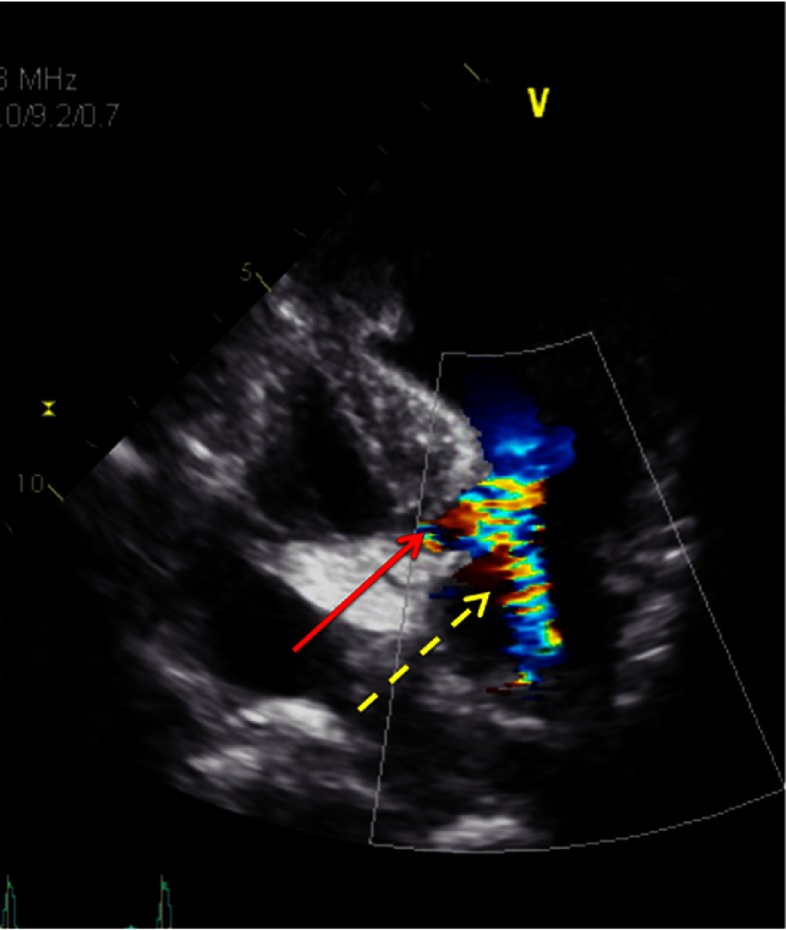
Echocardiography with Doppler showing severe mitral regurgitation and LVOT obstruction.
